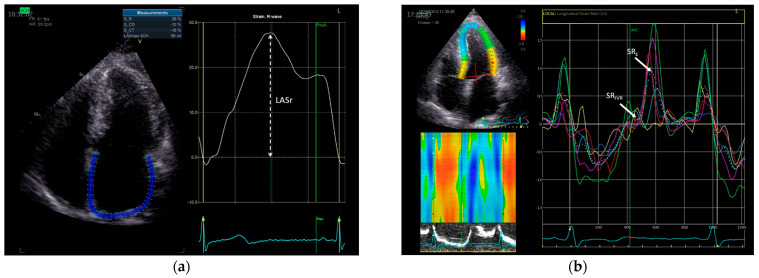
An example of (a) LA reservoir strain (LASr), and (b) LV global longitudinal strain during early diastole (SR_E_) and during the isovolumic relaxation period (SR_IVR_) in a patient with HFpEF. LA strain was determined as the average value of the longitudinal positive strain peak under LA relaxation from all six segments of the LA in the apical 4-chamber view, using the onset of the QRS as the referent point. The white dotted curve represents the average value of LA strain from all analyzed LA segments. SR_E_ and SR_IVR_ were determined as the average values of the longitudinal positive strain rate peak during LV early diastole and isovolumic relaxation period, respectively, from all LV segments in the apical 4-chamber view. Different color lines represent strain rate curves of individual LV segments. The white dotted curve corresponds to the average value of LV strain rate from all six analyzed LV segments. LA, left atrial; LV, left ventricular.
